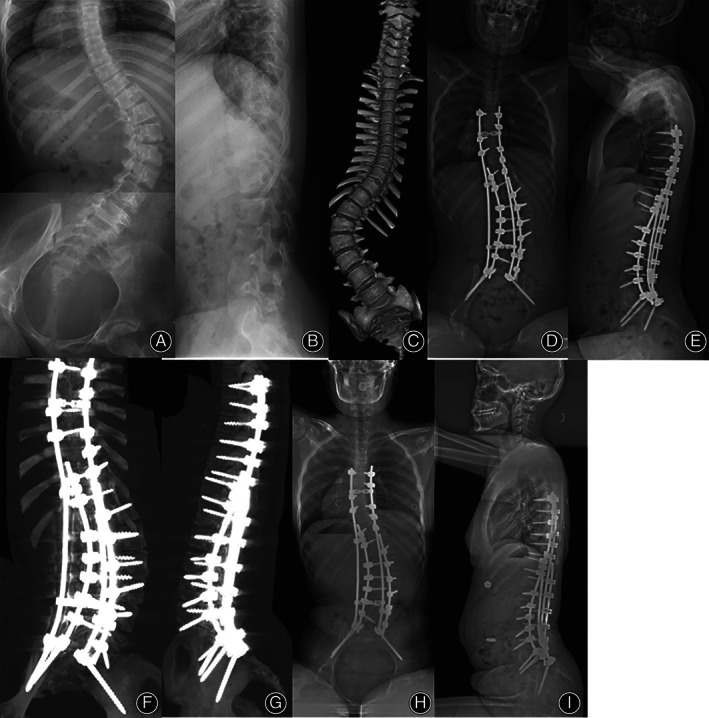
Female, 12 years old, diagnosed with osteopsathyrosis. (A, B, C) The pre‐operative anteroposterior radiograph and CT scans showed that Cobb angle was 90.4°, SPO was 30.7° and CB was 36.4 mm. (D, E) Postoperative anteroposterior radiograph showed significant correction of kyphoscoliosis and PO, the Cobb angle was 34.2°, SPO was 12.1° and CB was 9.3 mm. (F, G) Post‐operative CT scans showed the placement of unilateral dual S2AI screws. (H, I) 1‐year follow‐up, the anteroposterior radiograph showed the Cobb angle was 35.1°, SPO was 14.3° and CB was 6.2 mm, the correction maintained well.
